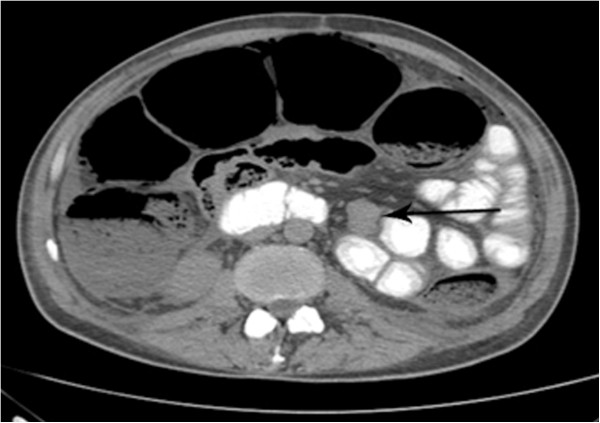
Abdominal CT shows hypodense nodular lesion next to the jejunum in the left flank, measuring 2.5 × 1.8 cm.
